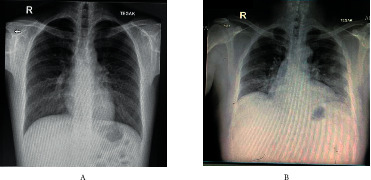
Chest X-rays of the patients. (a) Chest X-ray revealed a typical COVID-19 radiological appearance of diffuse infiltrate at the base, which extended to the periphery of the lungs. (b) Chest X-ray revealed a typical COVID-19 radiological appearance of basal nodular infiltrate at the periphery of the lungs, especially dominant in the left side.
